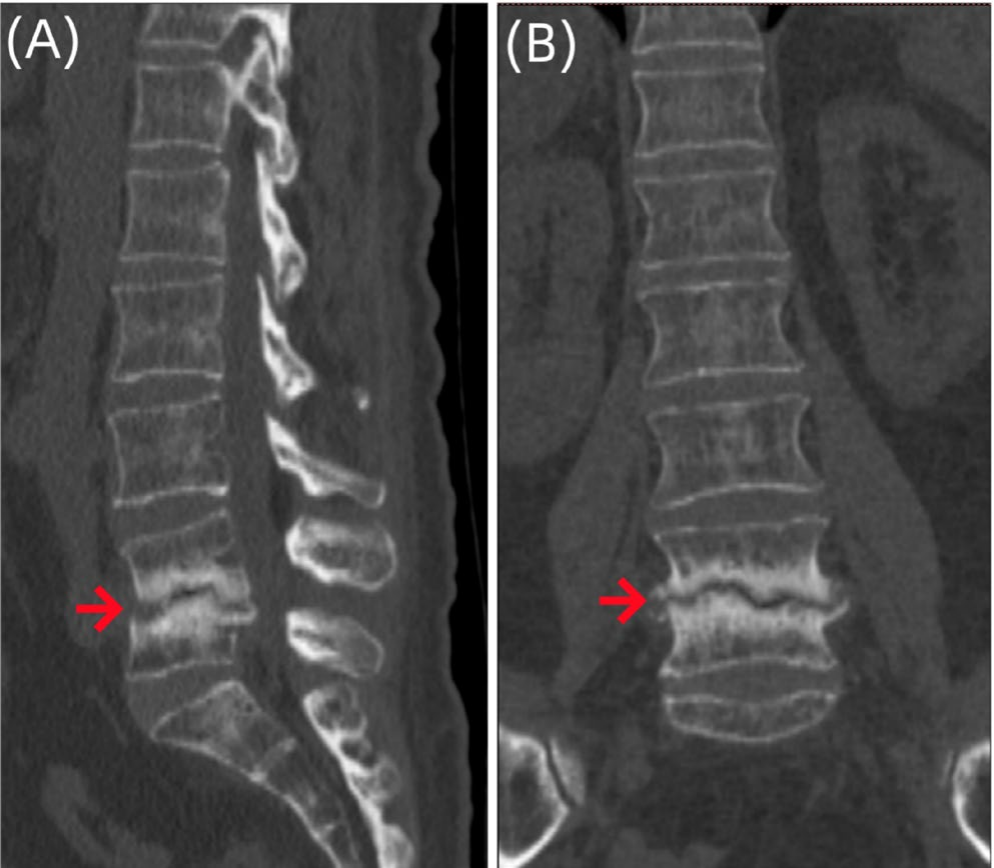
Three‐dimensional CT images of the lumbar vertebrae of the patient. Imaging examination showed that compared with Figure 6, there was no increase in bone destruction in L4 and L5 vertebrae, and the local bone mineral density was higher than before (A–B).
